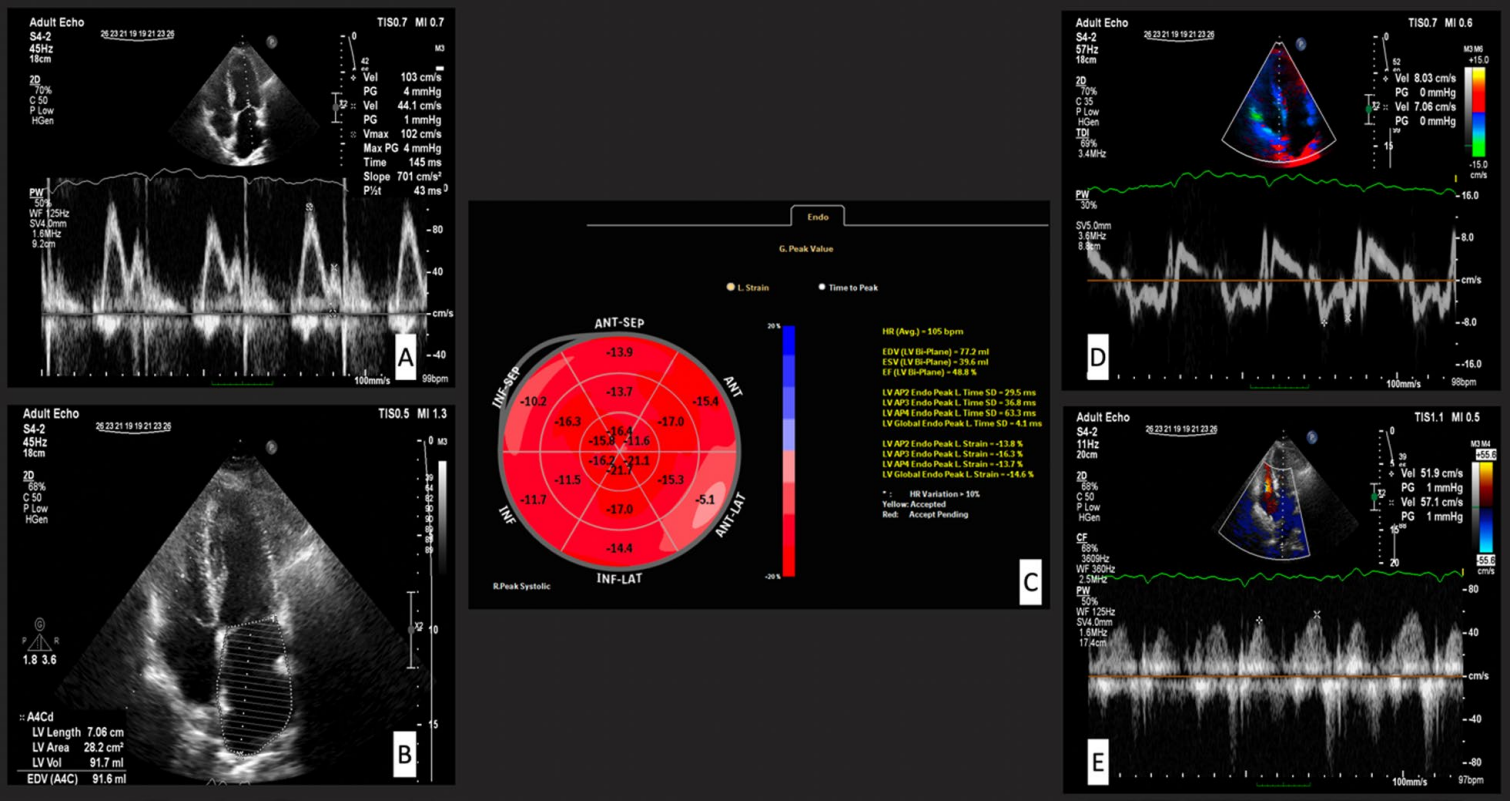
A Early mitral inflow velocity (*E*), Late mitral inflow velocity (*A*), and deceleration time (DT) by pulsed-wave Doppler at the tip of the mitral leaflets; B Left atrial area and volume in 4-chamber view; C Global longitudinal strain (GLS) of LV; D Mitral annular early diastolic velocity (*e*′) and late diastolic velocity (*a*′) of the septal wall from color-coded tissue Doppler imaging (TDI) image; E Pulmonary vein peak velocity in systole and diastole
